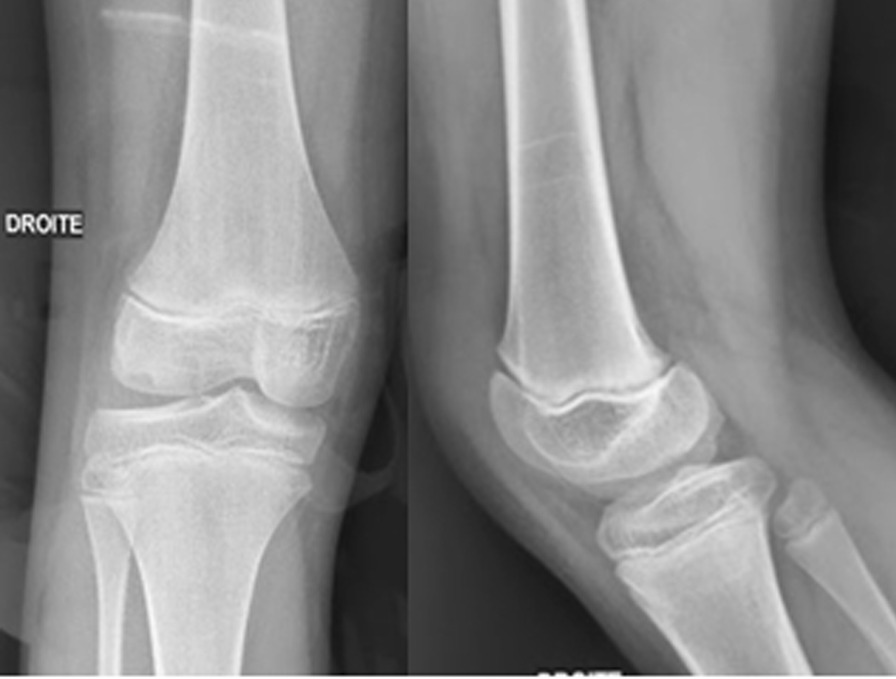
AP and lateral radiographs of the child’s right knee at the last follow up
